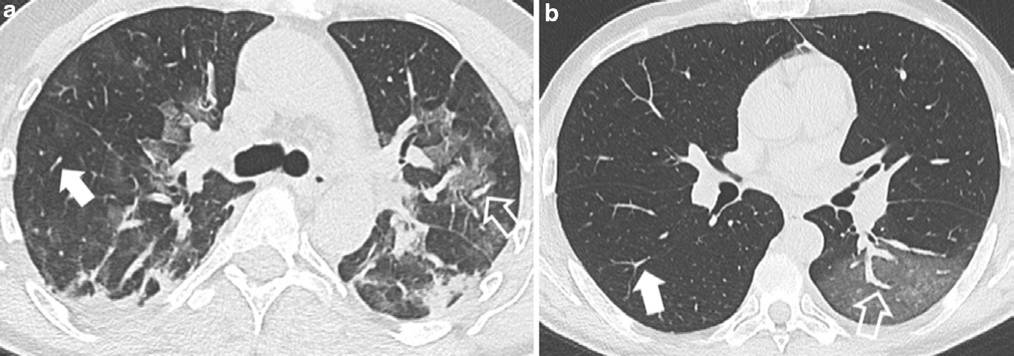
(a, b) Axial CT image without contrast shows enlarged pulmonary artery within ground-glass opacity (open arrow in a) compared with homologous vessel in the contralateral lung (solid arrow in a). Pulmonary vein within patchy area of ground glass opacity (open arrow in b) appears substantially enlarged compared with homologous vessel in the contralateral lung (solid arrow in b).
